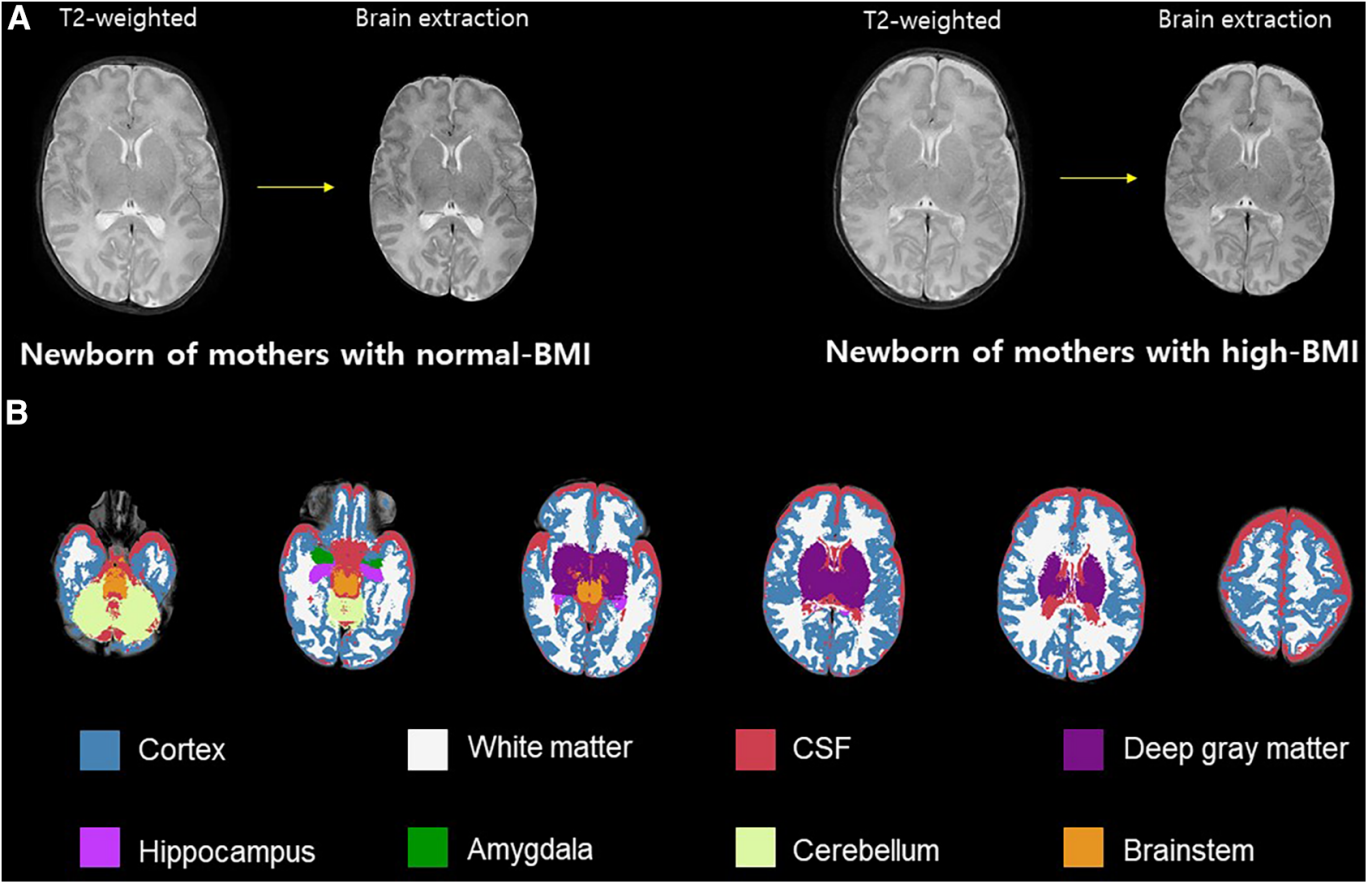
Volumetric analysis. Brain skull excluded from T2-weighted image (A). Example axial slices overlaid on T2-weighted images and segmented into eight brain morphological tissues (B). BMI, body mass index; CSF, cerebrospinal fluid.
